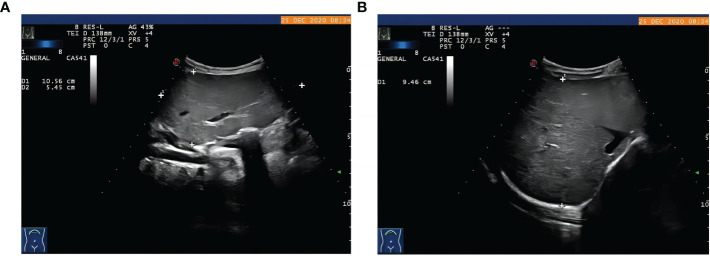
The proband with hepatomegaly. Abdominal ultrasound examination showed hepatomegaly with (A) the thickness of left liver lobe of 5.45 cm, vertical diameter of 10.56 cm, (B) right liver oblique diameter of 9.46 cm, normal echotexture, and no splenomegaly.
